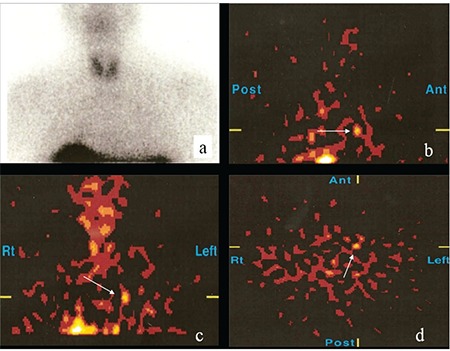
Planar and SPECT images of In-111 octreotide SRS of a patient with pulmonary carcinoid tumor. Although the planar image is negative (A), in sagittal (B), coronal (C) and axial (D) SPECT images the lesion can be seen
